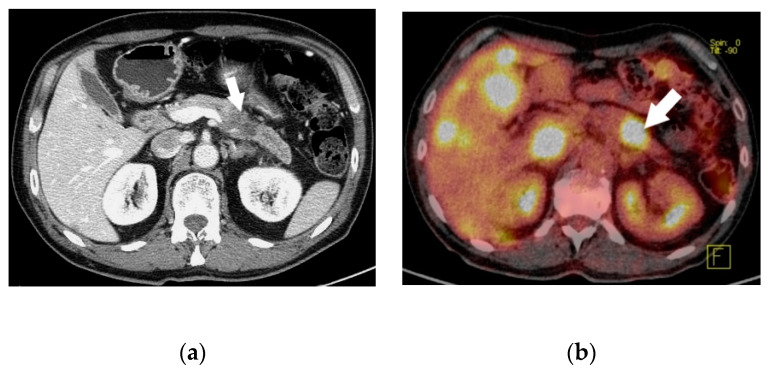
Computed tomography scan 2 (a) and ^18^F-FDG positron emission tomography/CT 2 (b) showing a pancreatic neuroendocrine tumor in the body of the pancreas (white arrow) with multiple liver metastases (case n.7).
